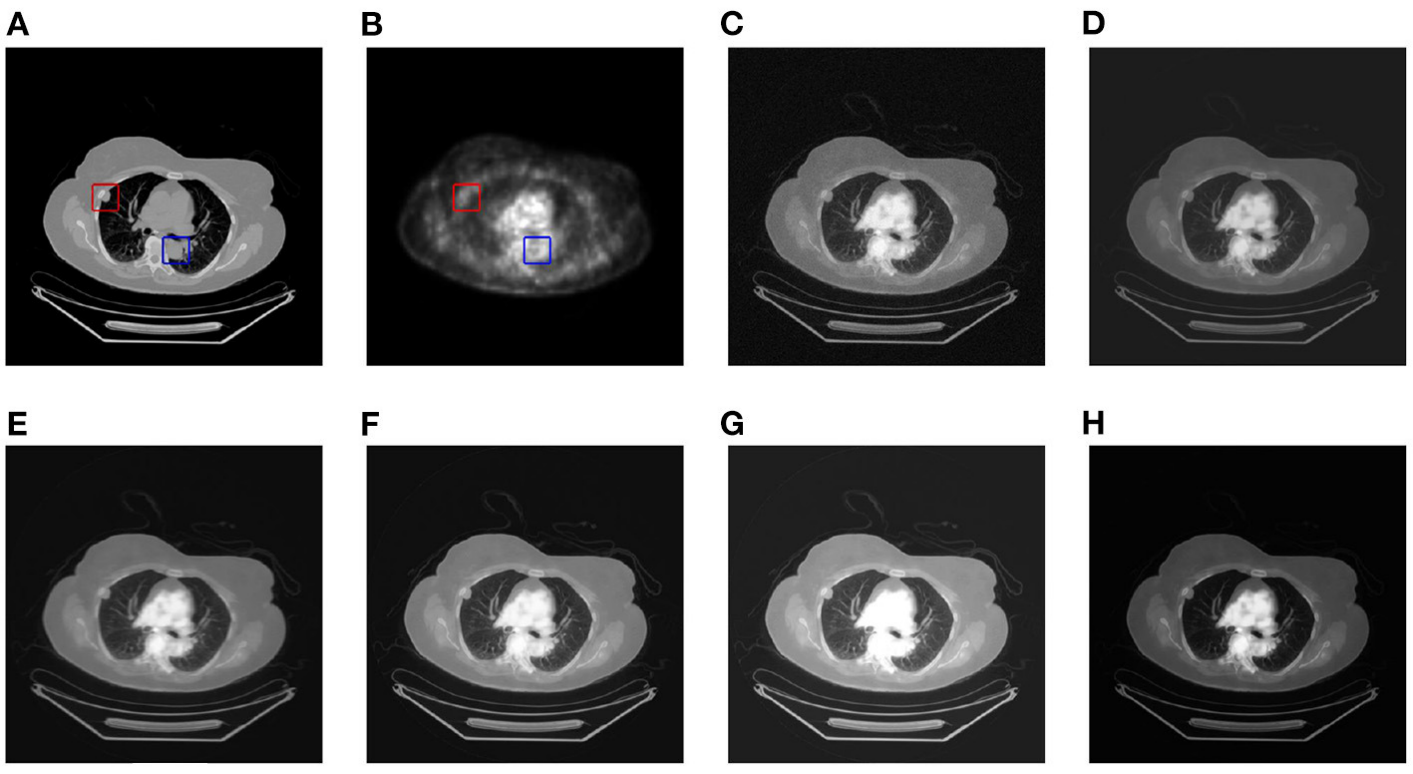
The qualitative comparison results of patient B. (A) CT; (B) PET; (C) AD; (D) GF; (E) PAPCNN; (F) MCFNET; (G) IFCNN; (H) OURS.
